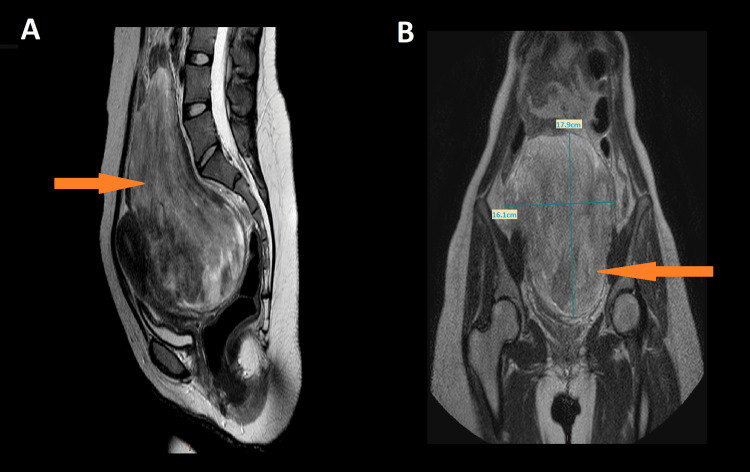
Magnetic resonance imaging of a young woman with uterine leiomyosarcoma (arrow) Sagital T2(A) and Coronal T2(B) show a large uterine mass measuring 18 x 16 x 11.5 cm, with hypointense and hyperintense signals on T2.
